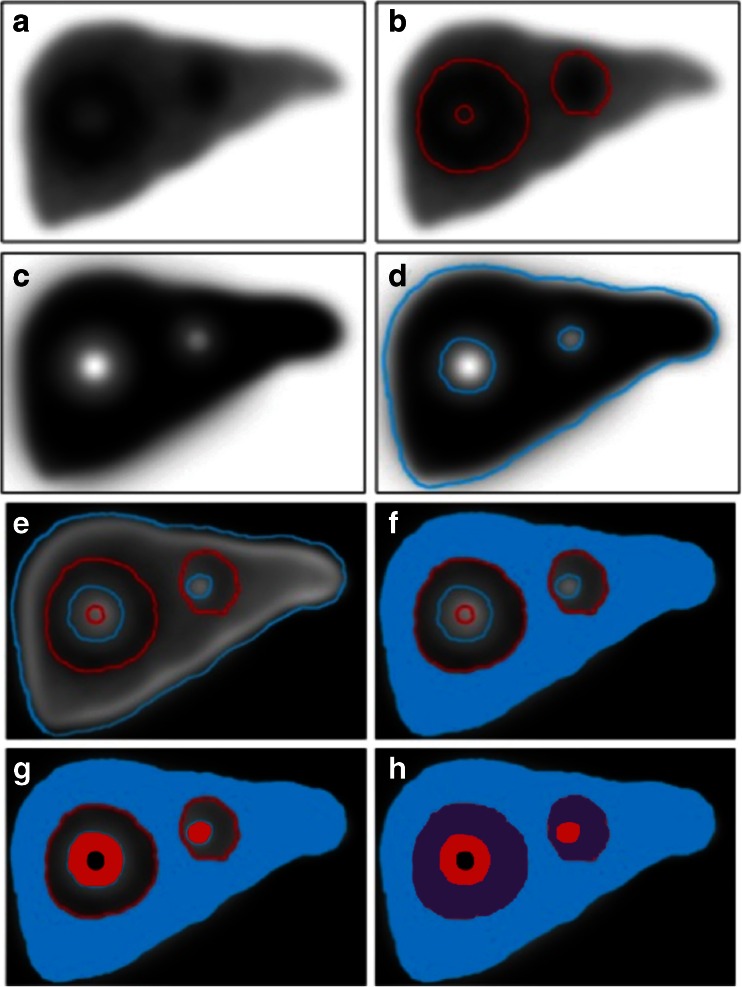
A threshold applied to the TcMAA SPECT image (a) defines the MAA-positive volume (b), and to the ^99m^Tc-sulphur colloid (SC) SPECT (c) defines the SC-positive volume (d). Coregistration of the two SPECT scans results in four compartments: f unirradiated functional liver (*V*
